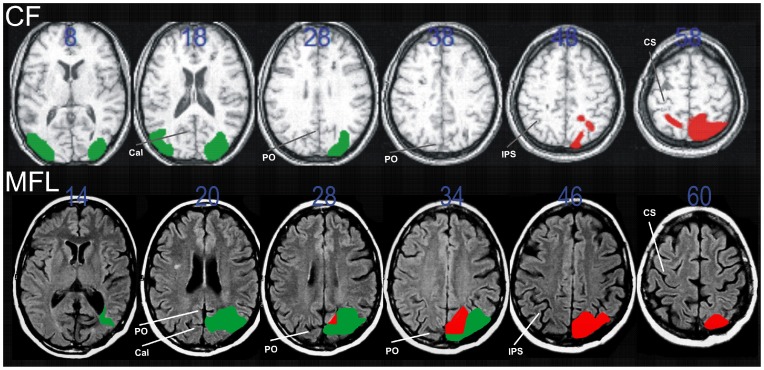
Anatomical MRI scan slices of patient CF (first row) and patient MFL (second row). The z-coordinates of the axial slices are indicated in blue. Occipital and parietal lesions were mapped and colored in green and red respectively. The major sulci are indicated to guide the localization of the lesions (Cal: calcarine, PO: parieto-occipital, IPS: intra-parietal, CS: central). Note that these MRI scans were acquired at the acute stage of the strokes and that at the time of testing no visual field defect was associated with the occipital lesions. The patients' lesions overlap to the greatest extent at the level of the right caudal superior parietal lobule, which is the pertinent anatomical substrate of their common chronic visuomotor deficits.
